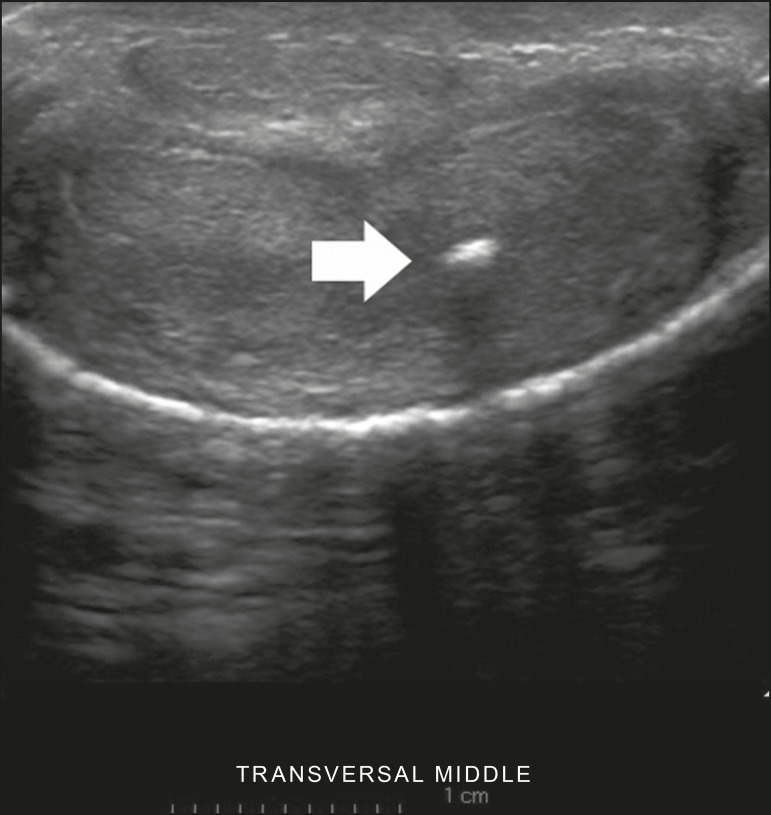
Transverse ultrasound of the penis, in a ventral view, in the middle
